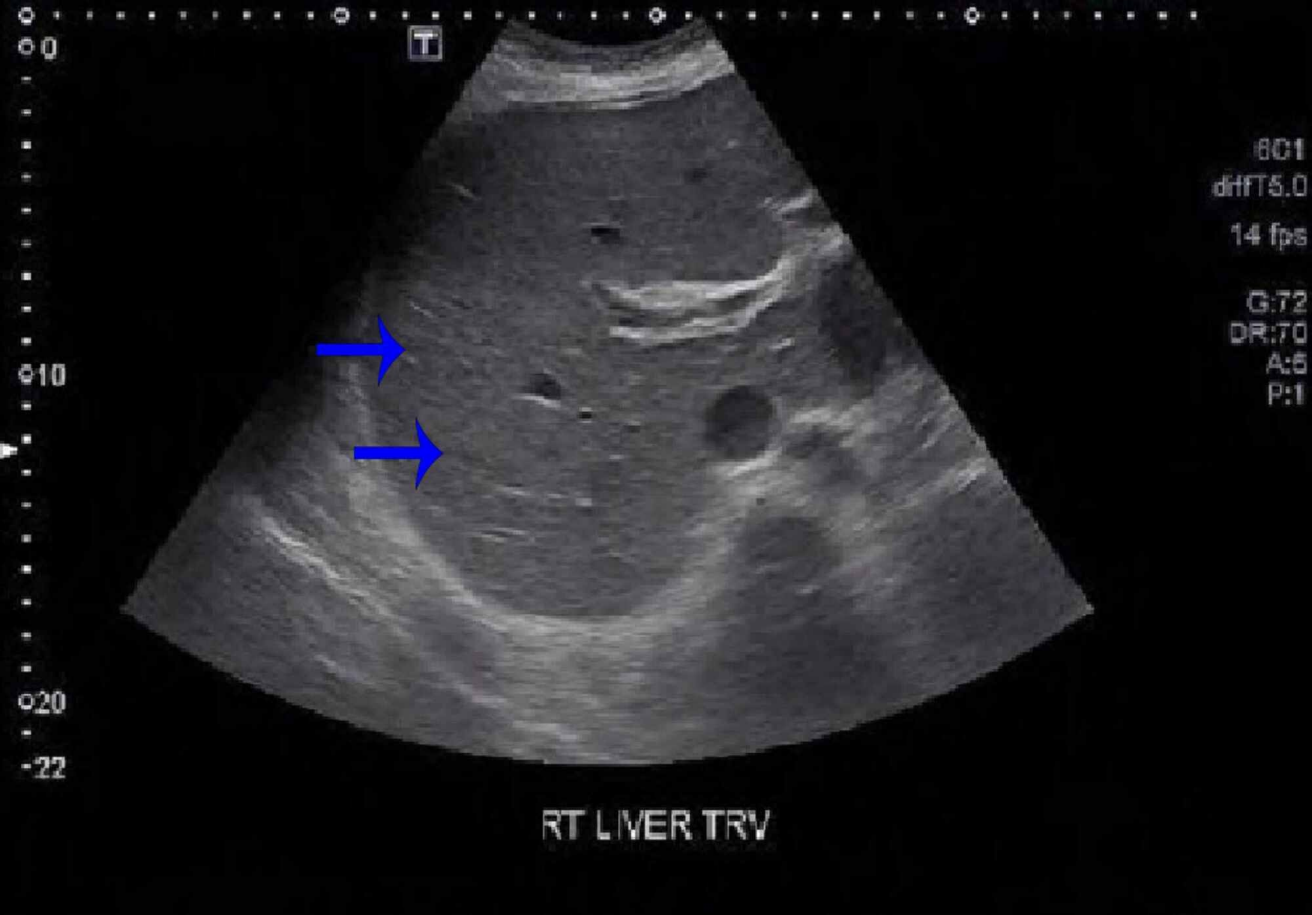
Abdominal ultrasound showing hepatic parenchymal disease
